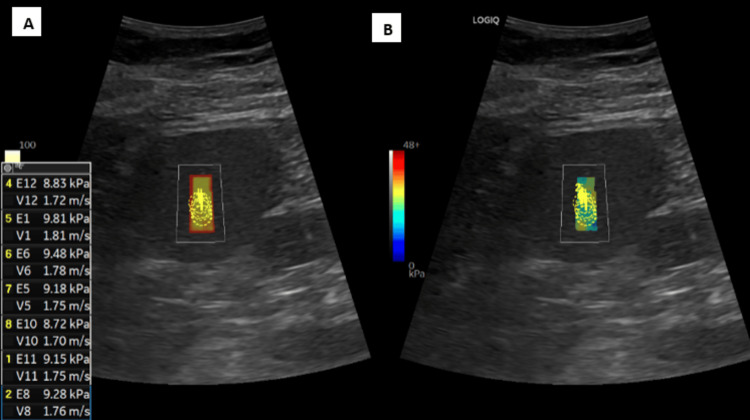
Ultrasound shear-wave elastography of the abdomen (A) B-mode grayscale image demonstrating a well-defined lesion in the splenic fossa. (B) Corresponding shear-wave elastography map demonstrating the same lesion with homogeneous stiffness distribution comparable to expected splenic tissue, without focal areas of increased stiffness or heterogeneity, supporting benign splenic tissue etiology and favoring splenosis.
